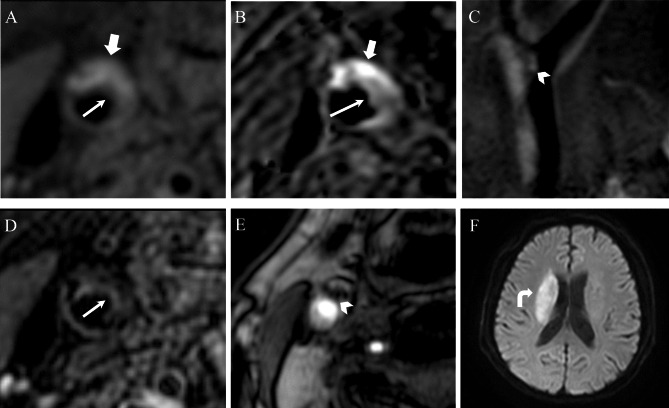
Patient, male, 70 years old. High resolution wall imaging of the internal carotid artery diagnosed vulnerable plaques in the right internal carotid artery with accompanying ipsilateral acute cerebral infarction. The following MRI images were obtained: (A) Axial T1WI clearly showed the plaque LRNC with hypersignal (fine arrow) and IPH with hypersignal (thick arrow);(B) Axial SNAP clearly showed that patch LRNC showed slightly hyposignal (thin arrow) and patch IPH showed significantly hypersignal (thick arrow);(C) Sagittal T1WI clearly showed a Ulcer with an irregular surface mixed signal (arrowhead);(D) Axial T2WI clearly showed hyposignal of plaque LRNC (thin arrow);(E) Axial 2D-TOF MRA raw image showed a patch of irregular surface Ulcer hyposignal (arrowhead);(F)Craniocerebral axial DWI showed ACI with flaky hypersignal surrounding the right lateral ventricularl (curved arrow)
